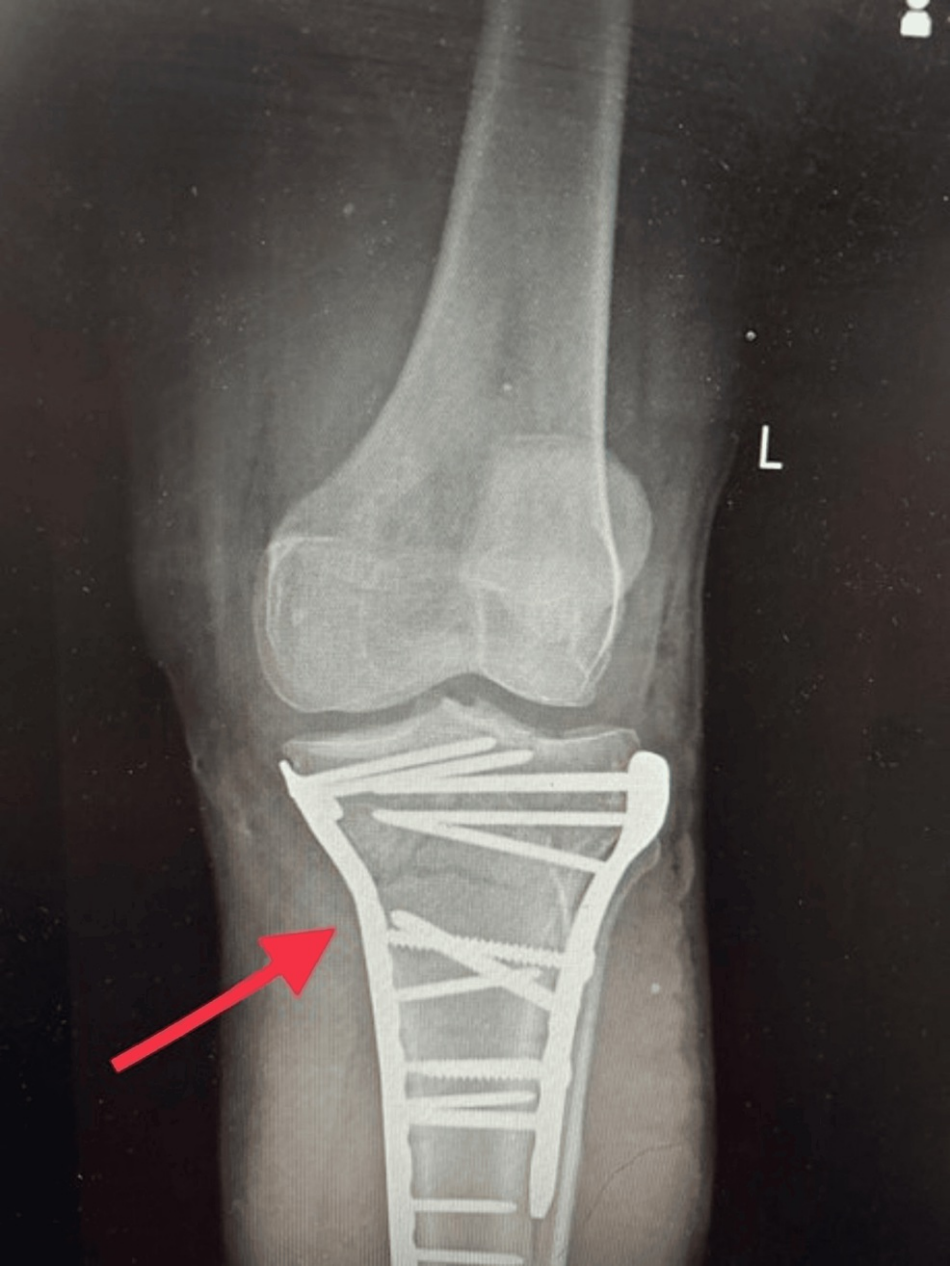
The post-operative radiograph of the left knee joint fixed with screws and plates
